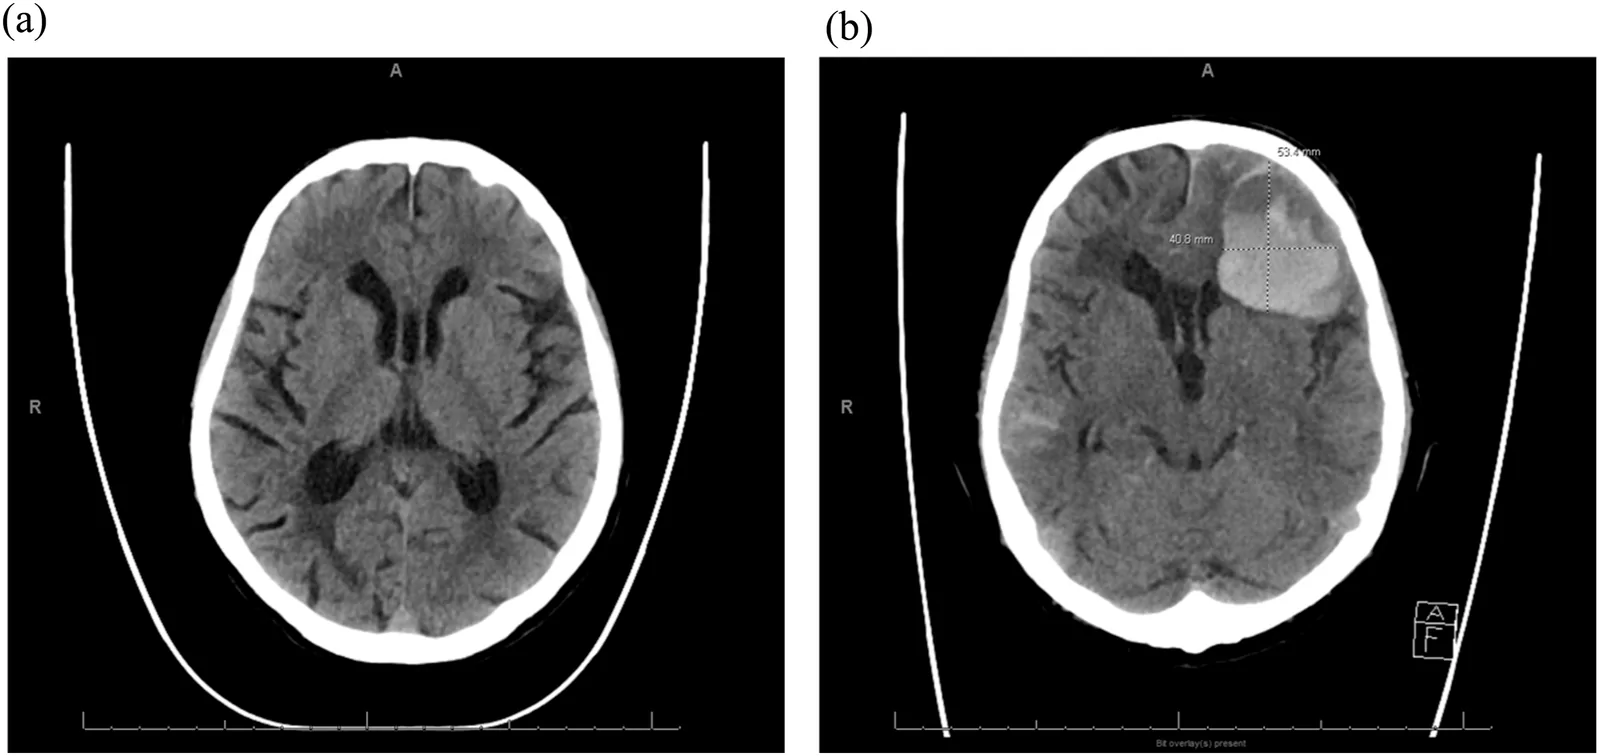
Head CT before and after tenecteplase administration. (A) Pre-thrombolysis noncontrast CT head obtained on 11/21/2025 10:21 demonstrated no acute intracranial hemorrhage, territorial infarct, extra-axial collection, or mass effect. (B) Post-thrombolysis noncontrast CT head obtained on 11/22/2025 demonstrated a large acute left frontal intraparenchymal hematoma, an additional right posterior parietal intraparenchymal hematoma, bilateral subarachnoid hemorrhage, intraventricular blood products, and associated rightward midline shift with subfalcine herniation
